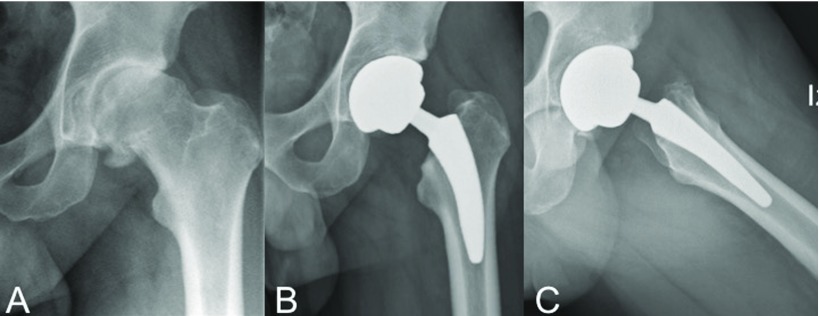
A Preoperative anteroposterior radiographs of a 43-yeard old male´s left hip with diagnosis of primary osteoarthritis B and C. Immediate postoperative anteroposterior (A) and lateral (B) radiographs showing adecuate implant positioning after implanting a MiniHip™ stem.
